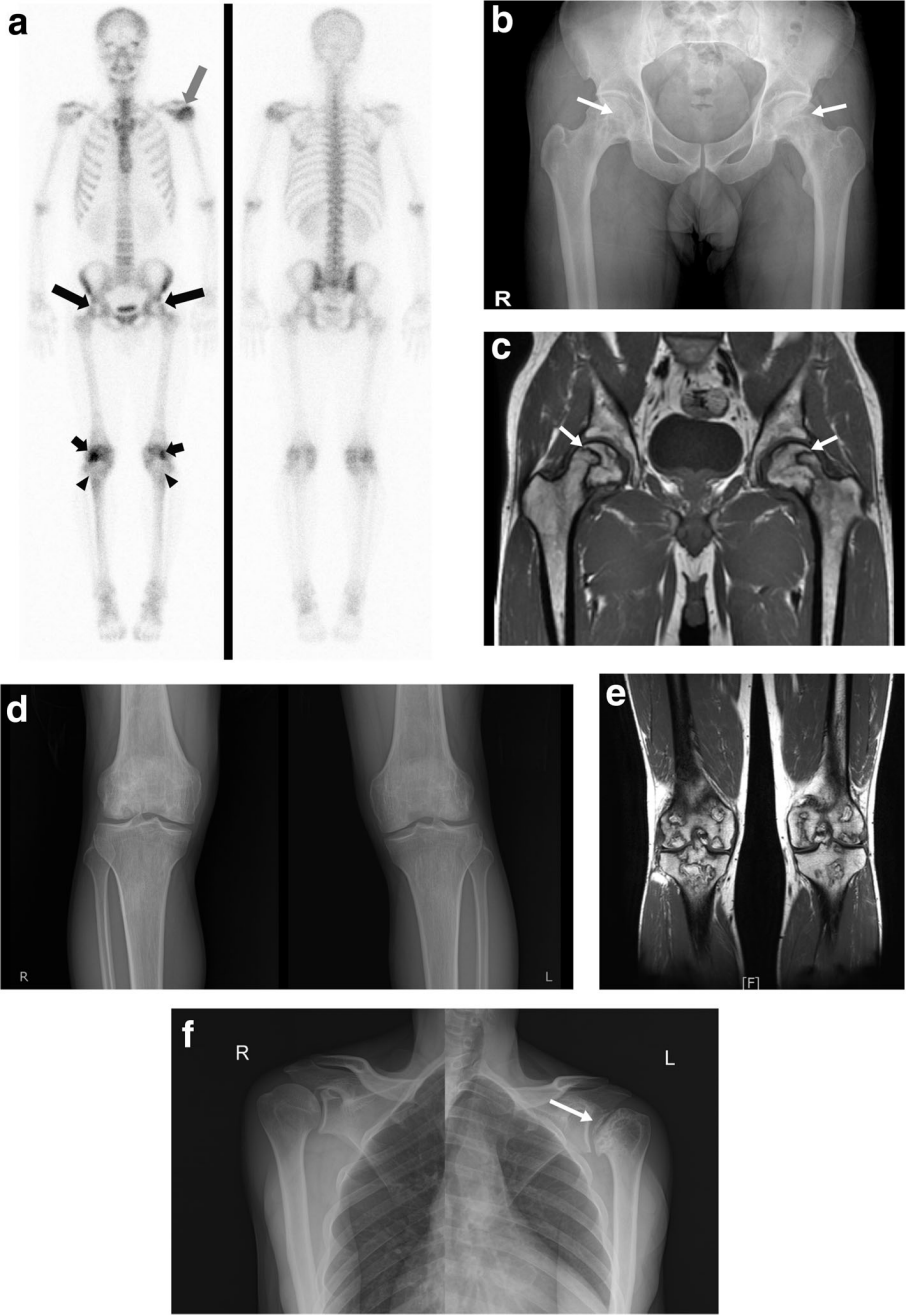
A 42-year-old male case of multifocal osteonecrosis (ON). a This is a bone scintigraphy performed in a 42-year-old male patient with abnormal photon-defects in both femoral heads (long arrows). Also abnormal hot uptakes were also observed in the left humeral head (white arrow), both distal femoral (short arrows) and proximal tibial regions (arrowheads), suggesting that AVN is suspected. b Pelvis anteroposterior view (AP) shows radiolucency and surrounding sclerosis of both femoral heads (arrows). c Coronal T1-weighted image shows large areas of low signal intensity demonstrated typical band-like pattern of ON involving both femoral heads (arrows). d Both knee AP views show ill-defined lucency and sclerosis with cyst like appearance of both distal femur and proximal tibia. e Coronal T1-weighted image shows multiple geographic lesions with peripheral low signal intensity of rim involving both distal femur and proximal tibia. f Simple radiographs show more advanced degree of ON with subchondral fracture and mild osteoarthritic change involving left humeral head (arrow)
